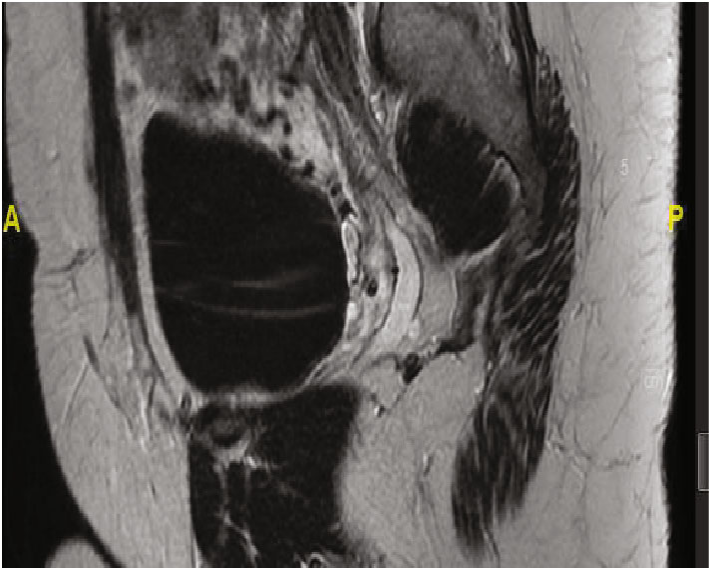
MRI imaging with radiology report eccentric intrauterine pregnancy associated with the c-section scar, extending into the posterior-lateral left adnexa where the placenta is uncovered from the myometrium. The placenta abuts the bladder without evidence for direct invasion.
